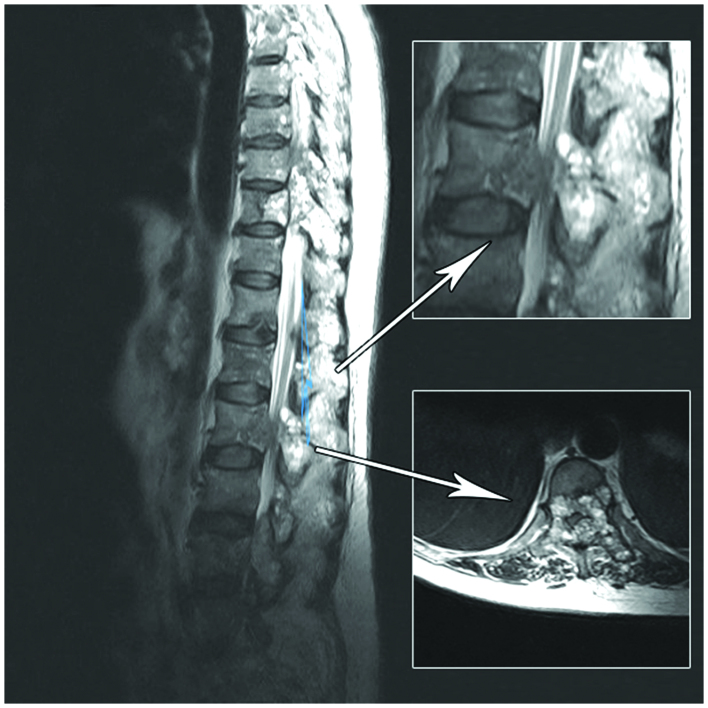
Magnetic resonance imaging of the vertebrae, demonstrating numerous abnormal vertebral bodies with marrow infiltration, particularly in the second lumbar vertebra. Upper and lower (inset) images indicate the sagittal and coronal planes, respectively.
